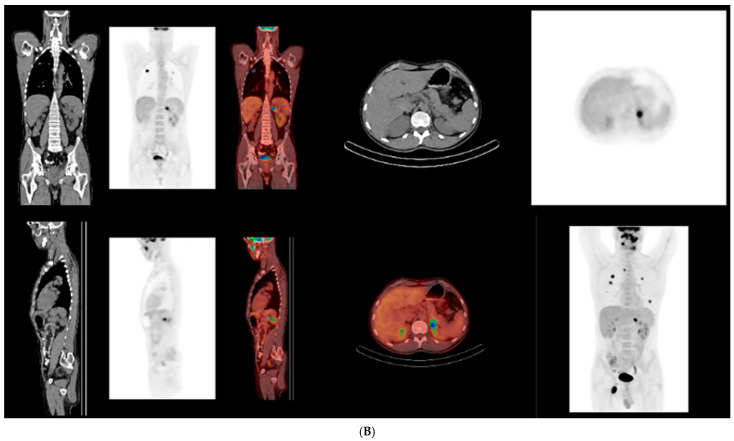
(A,B) ^18F-FDG PET–CT images obtained at the time of systemic relapse, demonstrating disseminated metabolically active disease involving nodal and extranodal sites.
